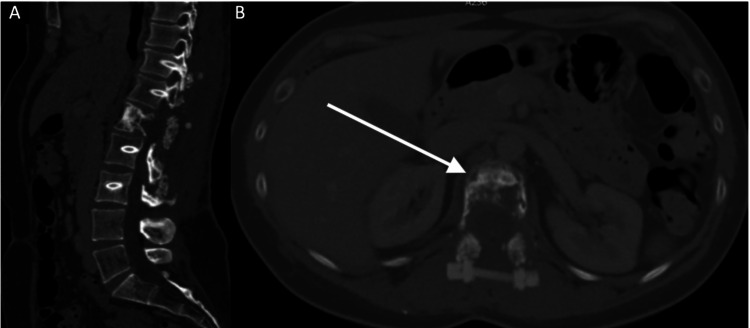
Postoperative computed tomography scan obtained 10 months following the stereotactic radiotherapy. A: sagittal reconstruction showing the calcified ventral aspect of the vertebral body L1 and no active metastatic disease. B: axial cut at the level of L1 showing calcification with the irradiated tumour and carbon-fibre implants. Note the complete lack of artefacts.
